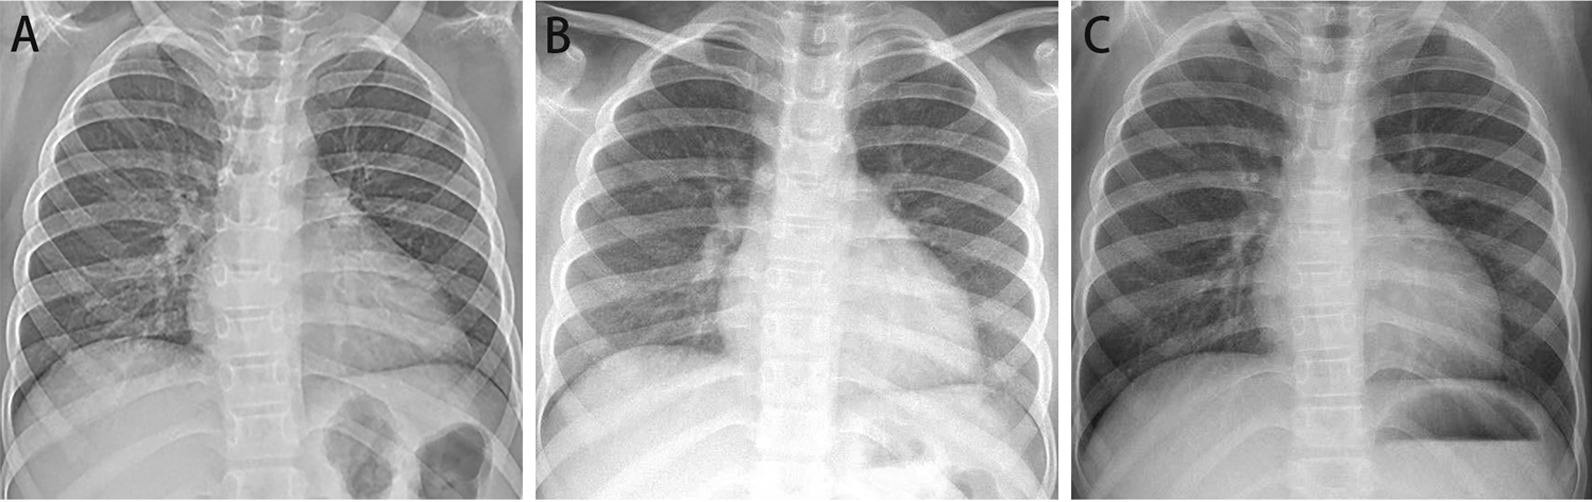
Chest radiography performed at 1, 15 and 21 months after discharge. The pulmonary lesions were significantly improved
